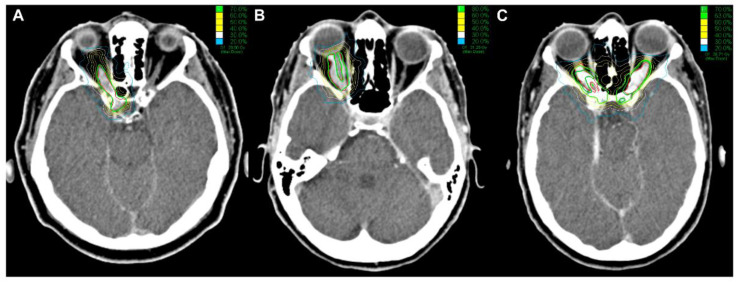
Cyberknife treatment planning showing axial planning-CT of a: (A) right sided ONSM without vision treated with 1 × 14 Gy (70% isodose); (B) right sided ONSM treated with 5 × 5 Gy (80% isodose) for vision preservation; (C) bilateral ONSM treated with 5 × 5 Gy (70% isodose) for the left and 5 × 4.5 Gy for the right side (red lines: PTV, green lines: prescription isodose).
